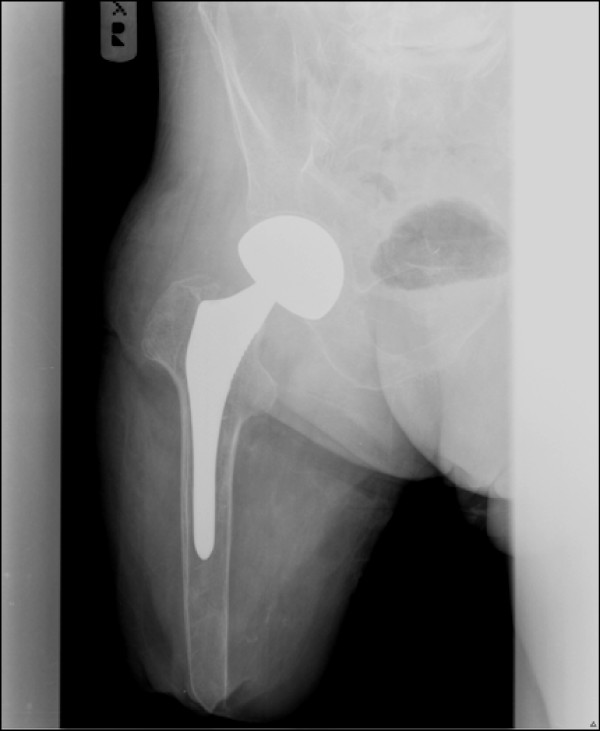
An xray five years after the hemiarthroplasty.
